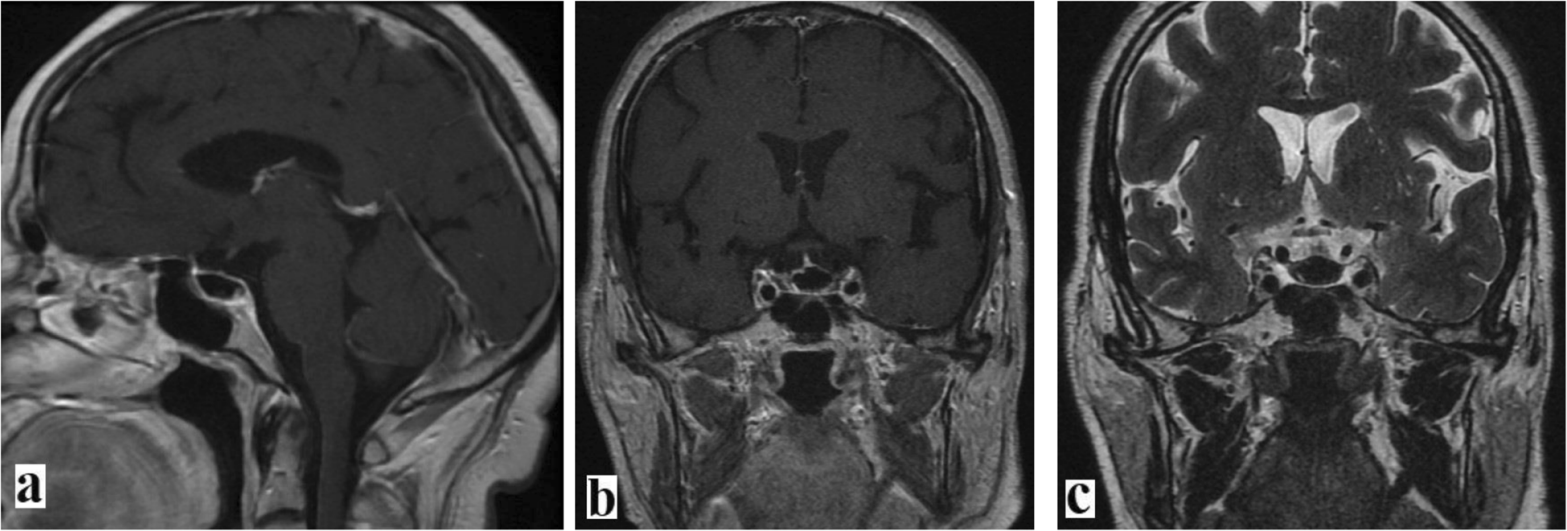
Same patient as in Fig. 3. Follow-up magnetic resonance imaging 3 months after the treatment showed complete drainage of the pituitary abscess, leaving as a sequela a cavity filled with air. a, b T1-weighted imaging + gadolinium. c T2-weighted imaging
